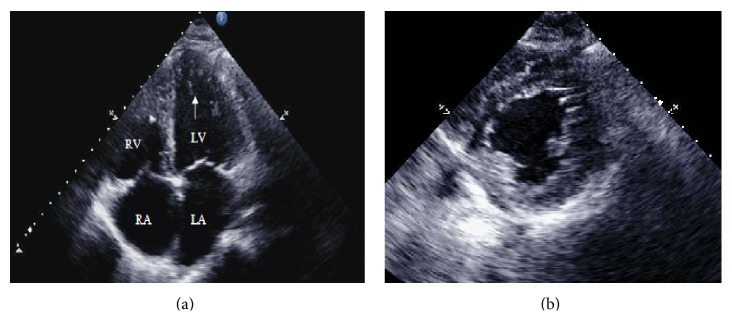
Echocardiogram of the heart. (a) Apical four-chamber view of the heart with marked trabeculations of the apex (white arrow). (b) Parasternal short axis view at the level of the papillary muscles with prominent LV inferolateral trabeculations. RA: right atrium, LA: left atrium, RV: right ventricle, and LV: left ventricle.
